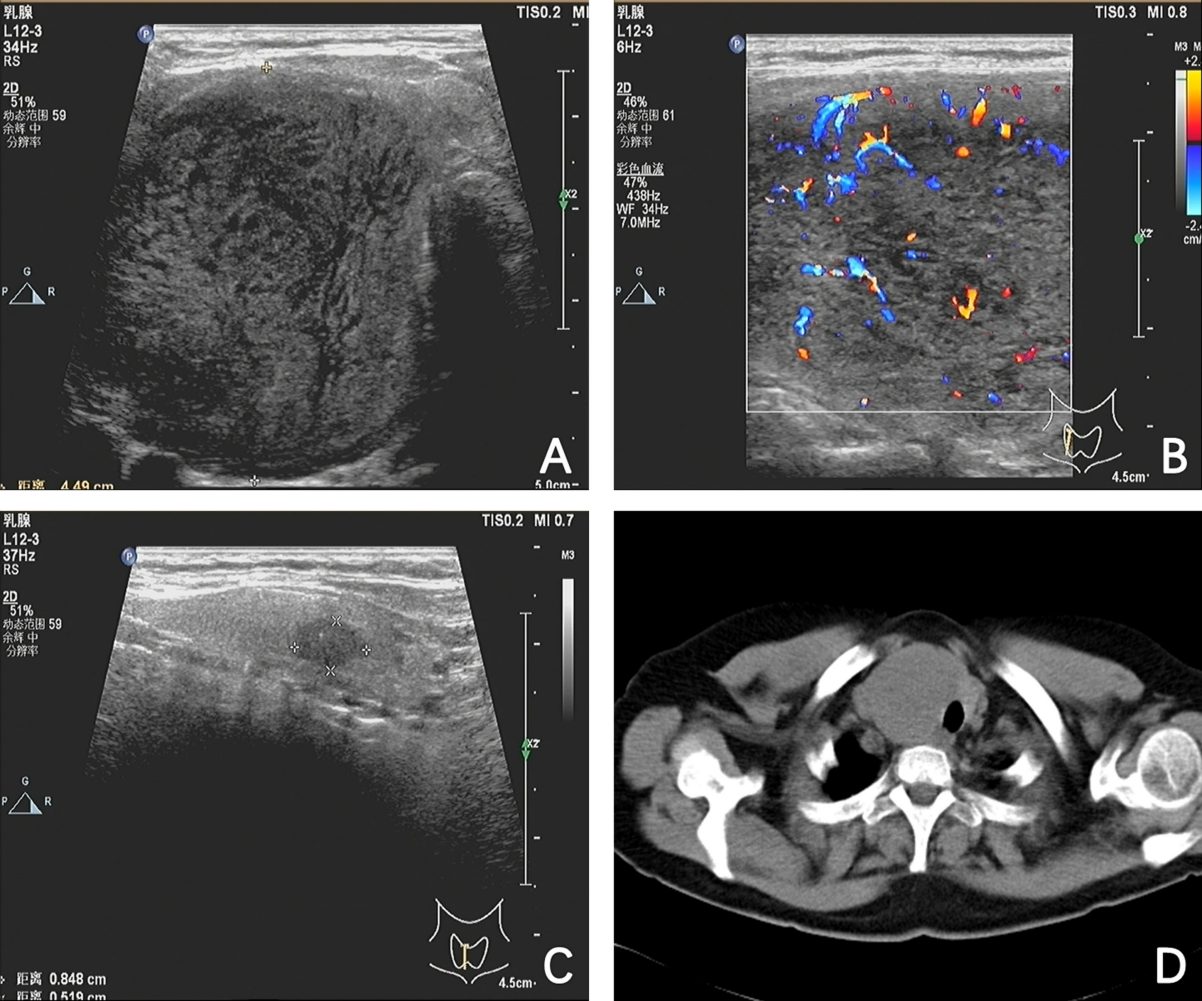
Ultrasound and computed tomography (CT) images of a tumor. (A) A hypoechoic mass measuring 65.4 mm × 34.1 mm × 57.5 mm in the right lobe. (B) The hypoechoic mass with rich blood flow signals in the right lobe. (C) A hypoechoic nodule with microcalcifications in the isthmus lobe. (D) A CT scan of the neck revealed an uneven thyroid gland with a slightly low-density mass in the isthmus and right lobe, ranging approximately 5.3 cm × 4.3 cm.
